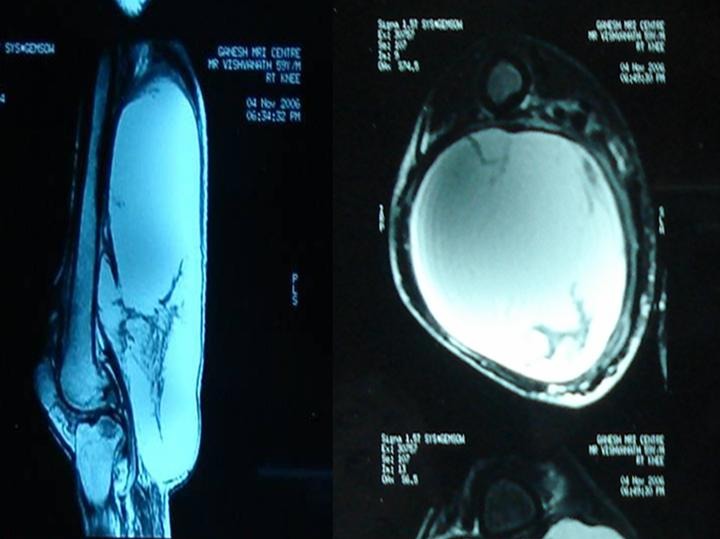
Sagittal and transverse section of right thigh showing large abscess on magnetic resonance imaging (MRI)
